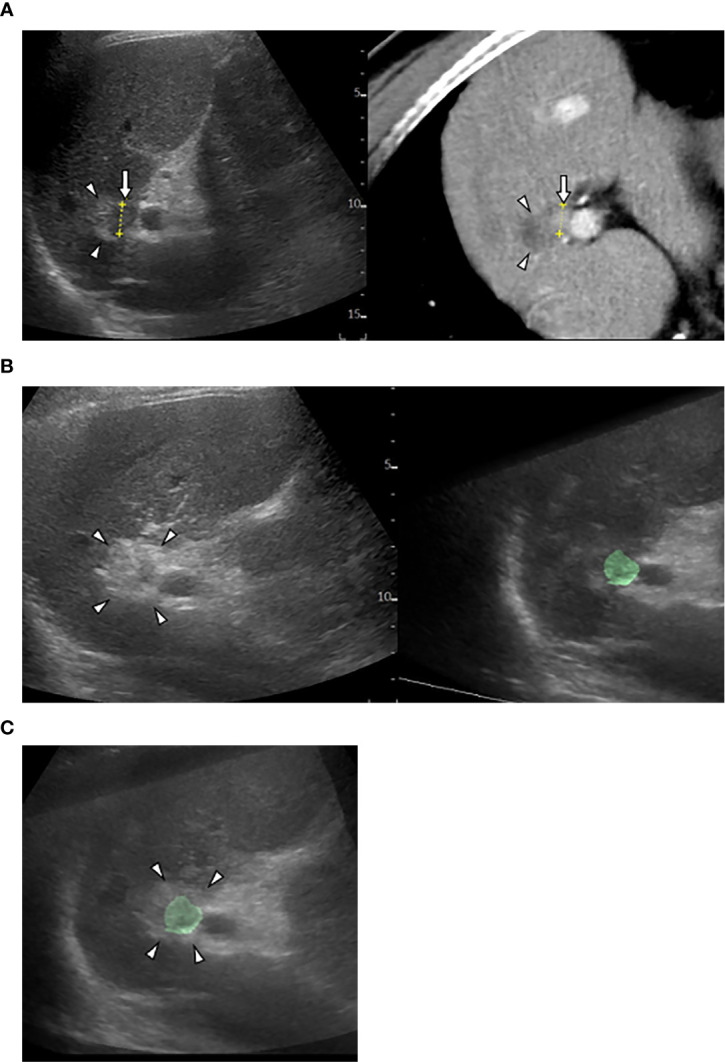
US-US fusion imaging and US-US overlay fusion. (A) CT-US fused image shows locally progressed hepatocellular carcinoma (HCC) (arrows) touching ablative necrosis (arrow heads) due to previous ablation. (B) US-US fusion imaging displays HCC colorized as green before ablation (right) and ablative hyperechoic zone (arrowheads) due to the present ablaion (left). (C) US-US overlay fusion demonstrates the green colorized HCC inside the ablative hyperechoic zone concentrically.
